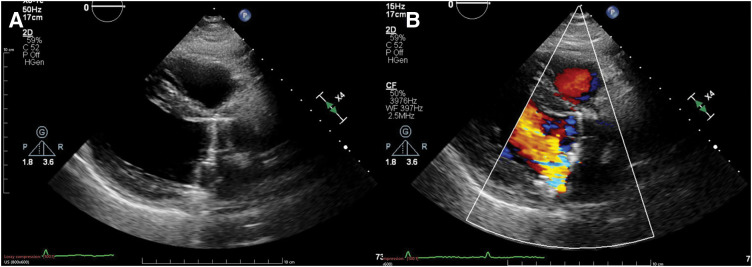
Follow-up TTE. Outpatient echocardiography reveals preserved valve function with complete closure of the abscess cavity. TTE, transthoracic echocardiography
